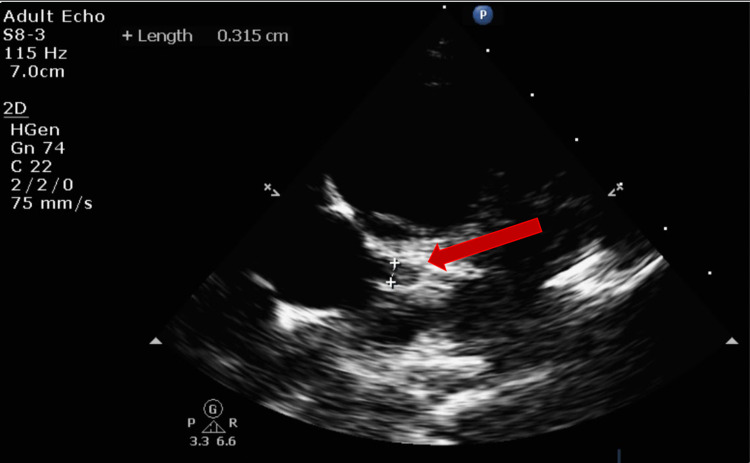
Transthoracic echocardiogram (TTE) showing left coronary prominence
